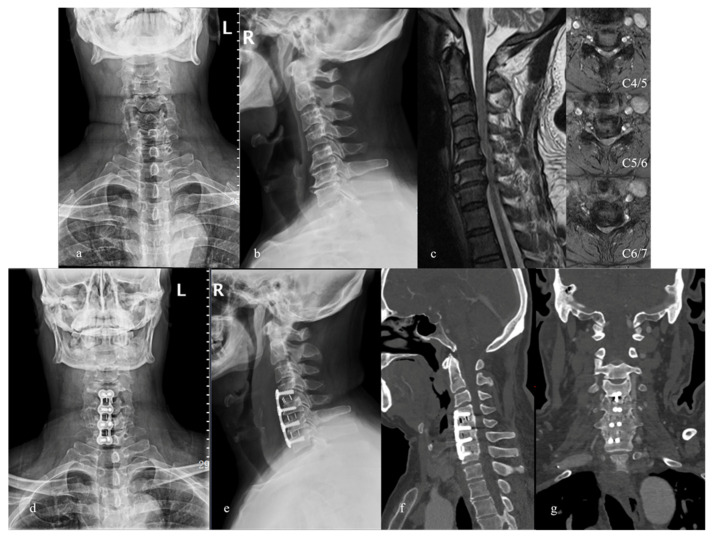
A 53-year-old male with upper limb numbness and clumsiness as well as walking disturbance for 1 year. (a,b) Preoperative X-ray films; (c) preoperative MRI showing spinal canal stenosis with C4-C7 disc herniation compressing the spinal cord; (d,e) postoperative X-ray films showing C4-C7 ACDF with plate-cage system; (f,g) CT scans at 3-year follow-up showing good implant position and bone fusion.
